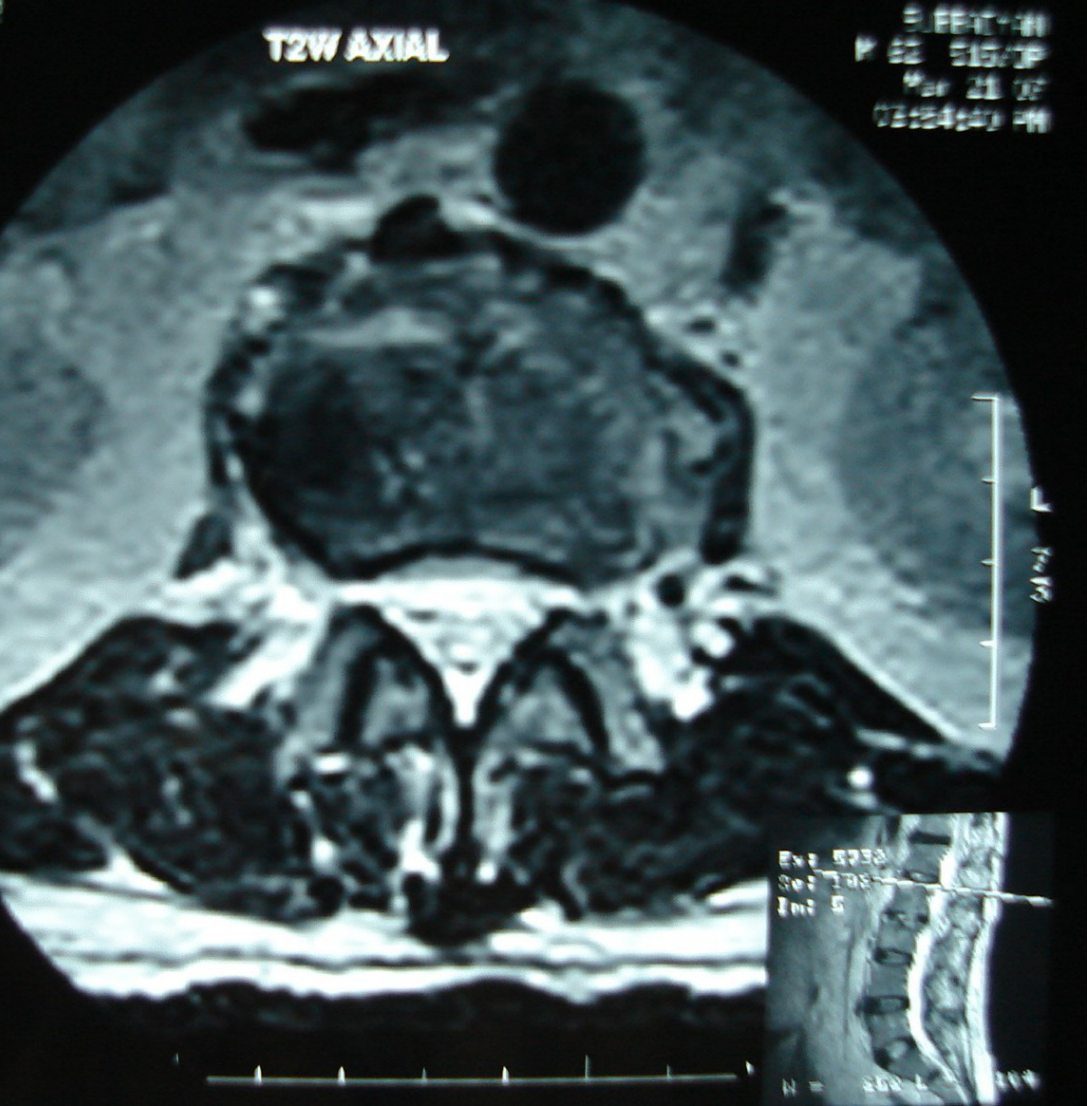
Axial T2 MRI images showing the above lesions
